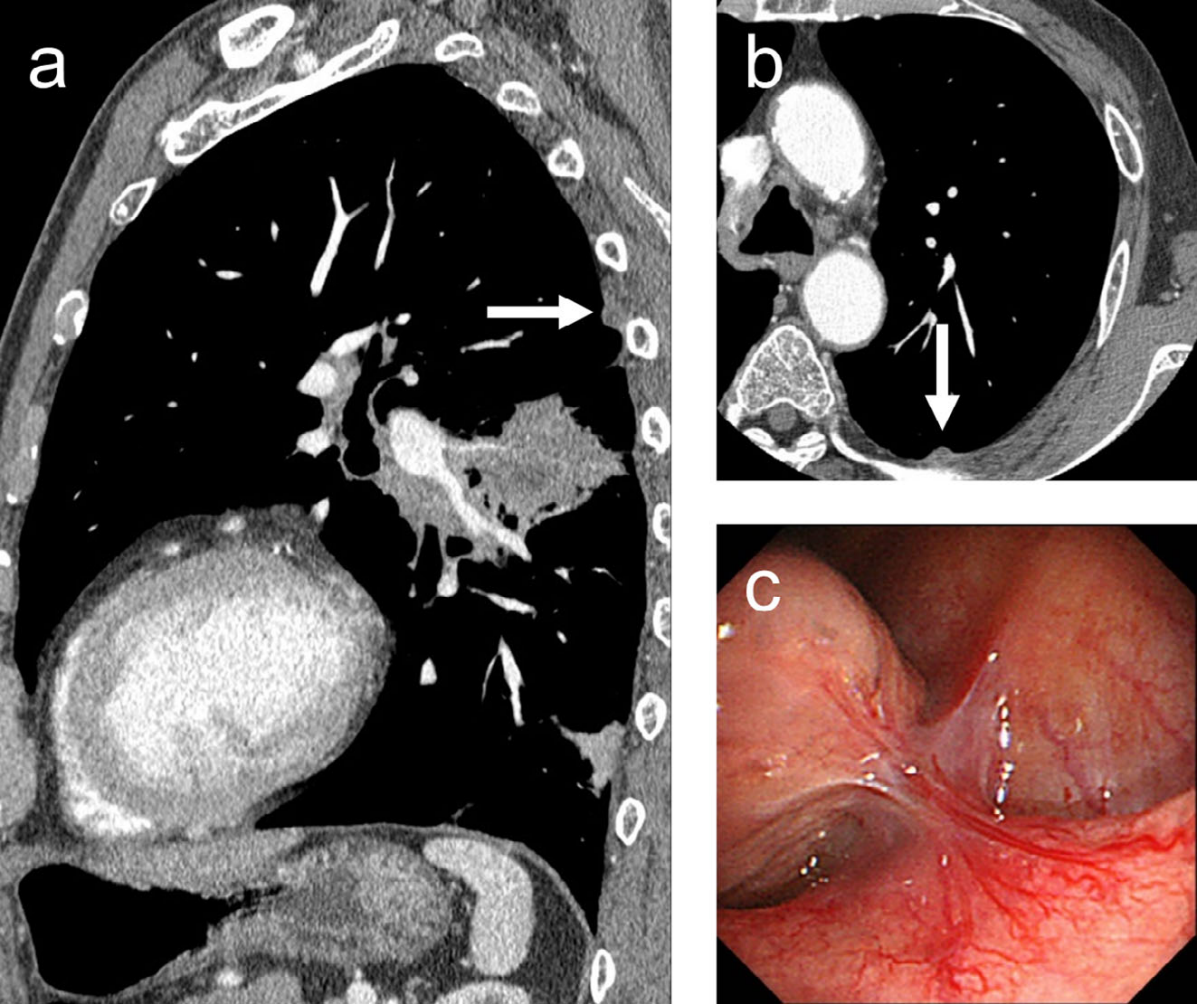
A case of failed pleural staging in a 78‐year‐old man (case 10 in Table 2). (a) Sagittal and (b) axial sections of a computed tomographic scan (mediastinal window) show a pleural nodule (arrow) on the head side of the primary lesion in the left lower lobe. (c) this nodule could not be identified on local anesthetic thoracoscopy because of the adhesion between the chest wall and lung
